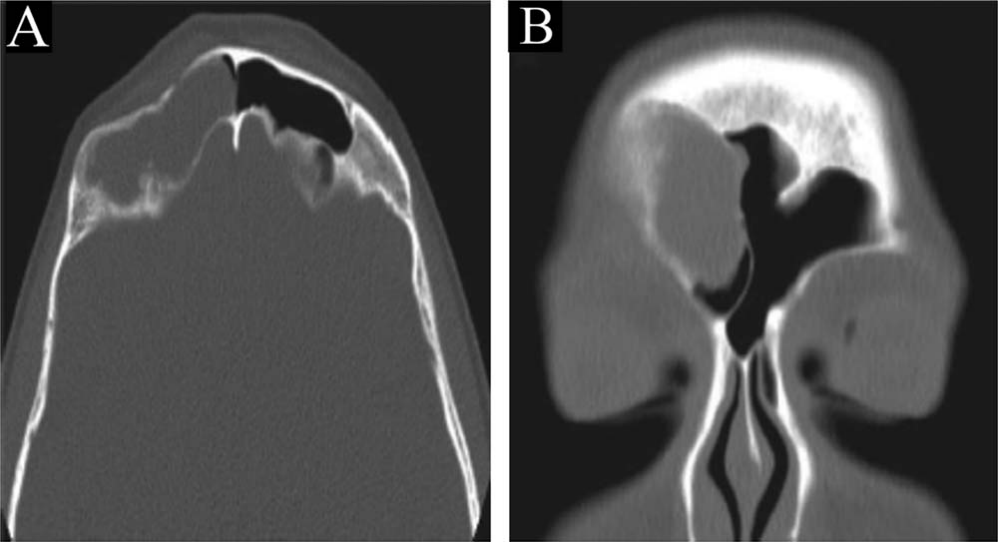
CT scan of the brain and paranasal sinuses in (A) axial and (B) coronal views, showing a lesion in the right frontal sinus with resorption of posterior and lateral walls. The anterior wall shows a depressed fracture.
